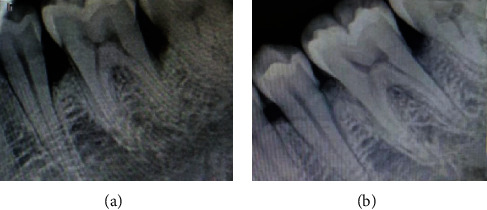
Radiographic image: (a) preoperative and (b) postoperative.
